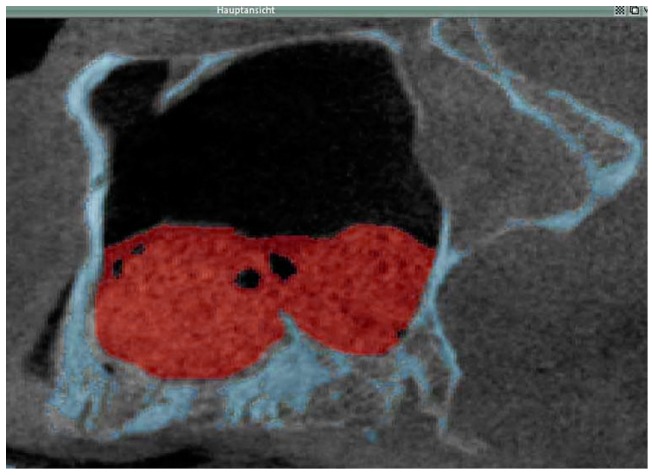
Two-dimensional radiographic image generated from the secondary cone beam CT acquired directly after SFA showing of a cross section through the augmented sinus floor (red) as well as the osseous anatomical structures of the native sinus floor (blue). This image was generated by merging the data sets of the primary and secondary cone-beam CTs. Sagittal view of the, left-sided maxillary sinus, patient 5.
